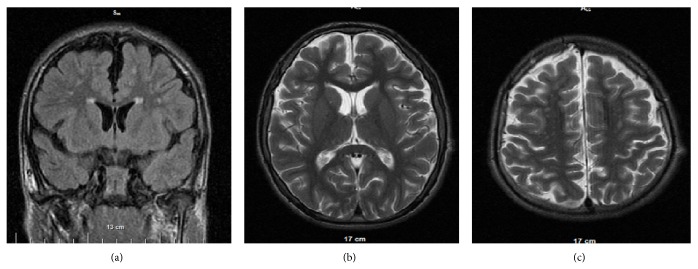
Patient number 7: a 13-year-old boy with severity score 7. (a) Coronal FLAIR image demonstrates dilated subarachnoid spaces, periventricular dense rim, and dense subcortical and periventricular foci in frontal lobes. (b), (c) Axial T2W image shows bilateral frontal subcortical hyperintensities (dense: 2 points), dilatation of subarachnoid spaces, and no significant dilatation of ventricular system (severity of global brain atrophy: 1 point).
